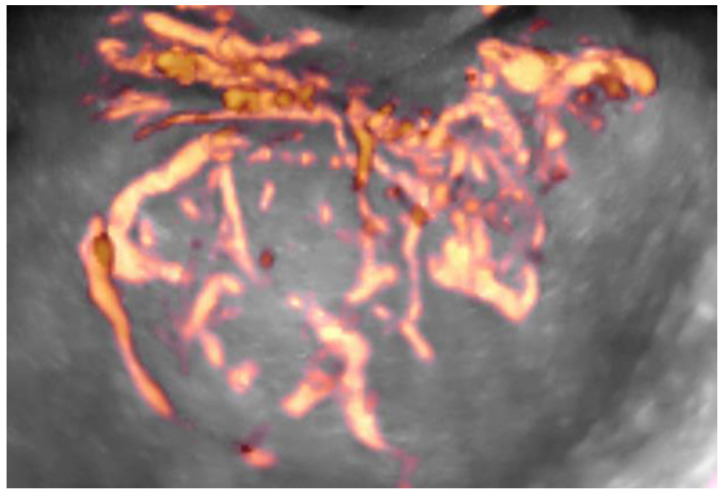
3D Power Doppler spatial image of the net of vessels (yellow-red color areas) supplying an ovarian tumor (Glass Body option).
